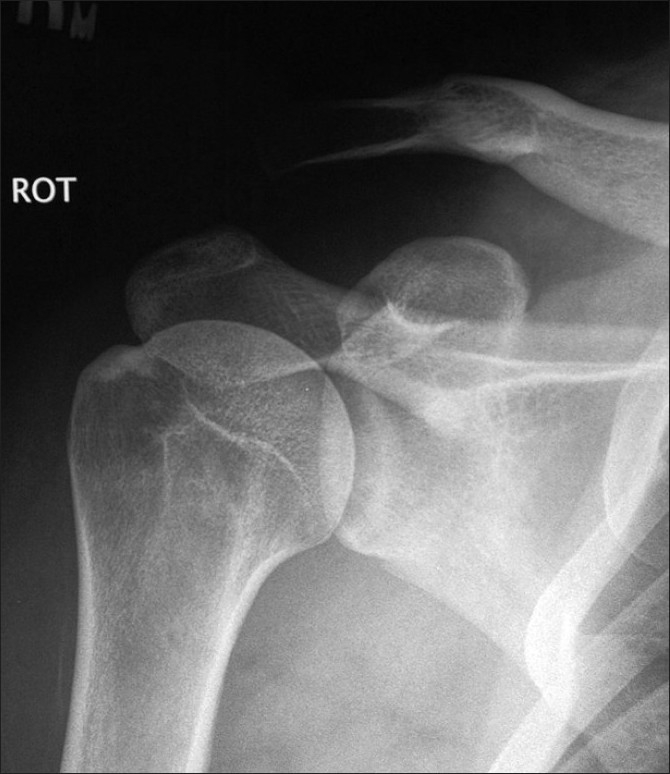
Radiograph of the shoulder showing type III dislocation of the acromioclavicular joint
